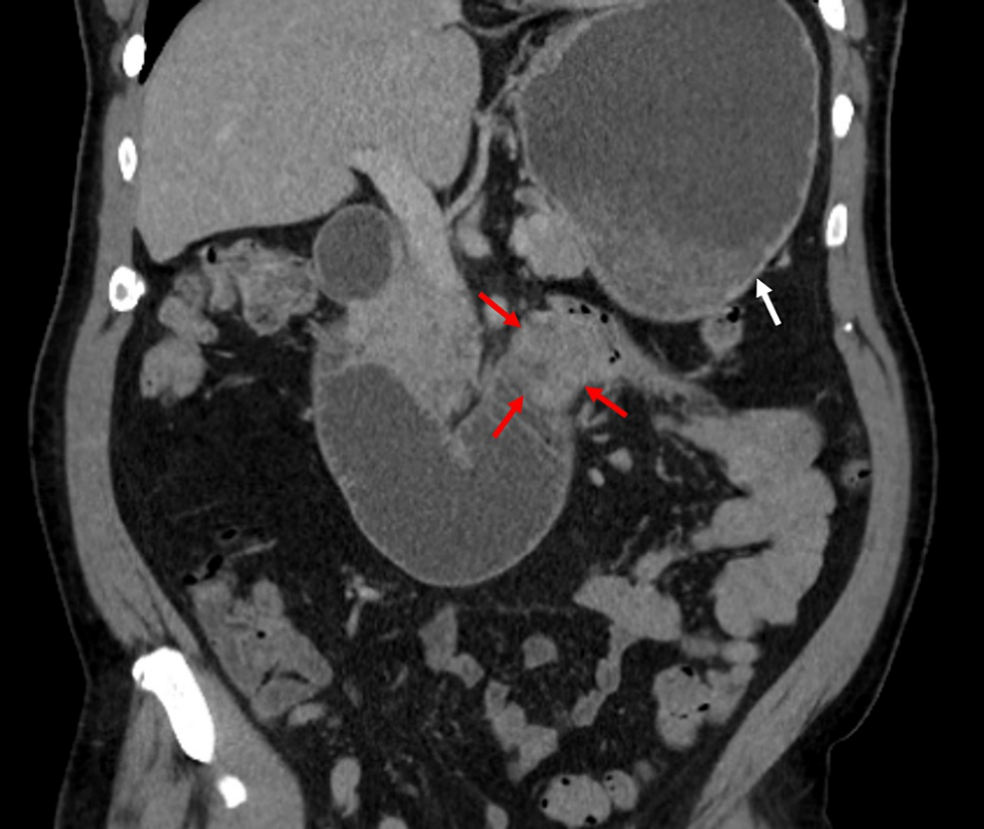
Contrast-enhanced CT scan of the abdomen demonstrating a soft tissue density at the duodenojejunal junction (red arrows) and a dilated stomach (white arrow).
